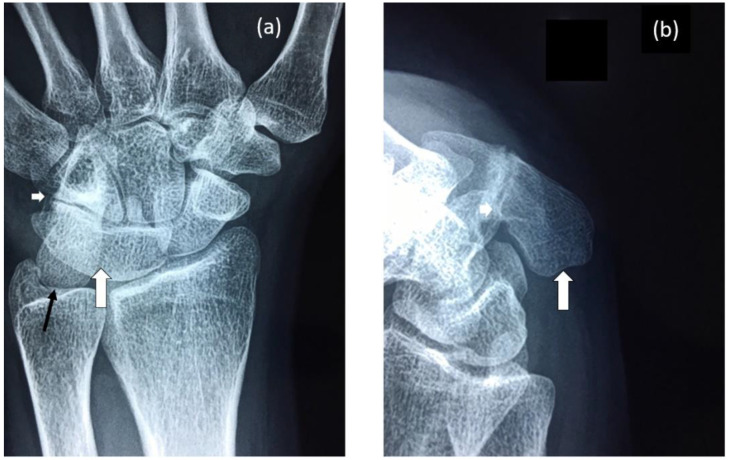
Radiographs of proband’s wrist highlighting: (a) Fusion of lunate and triquetrum (large white arrow), nearly complete fusion of hamate and pisiform (small white arrow) and elongation of the pisiform (black arrow). (b) Elongated pisiform (large white arrow) and fusion of hamate and pisiform (small white arrow).
